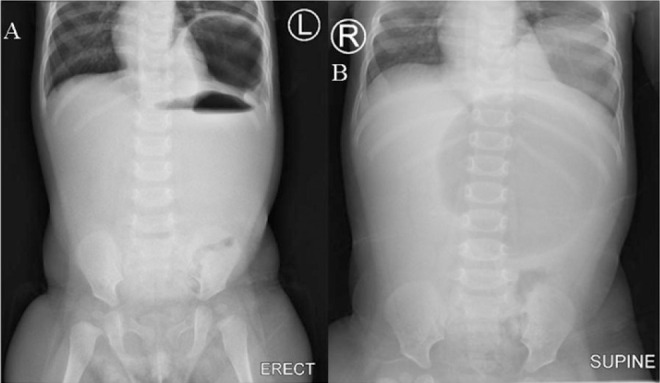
An Abdominal X-Ray showing Gastric Air-Fluid Levels A: Erect abdomen x-ray. B: Supine abdomen x-ray. X-rays demonstrate a left congenital diaphragmatic hernia with protrusion of the stomach into the left hemithorax. Findings suggestive of bochdalek hernia.
